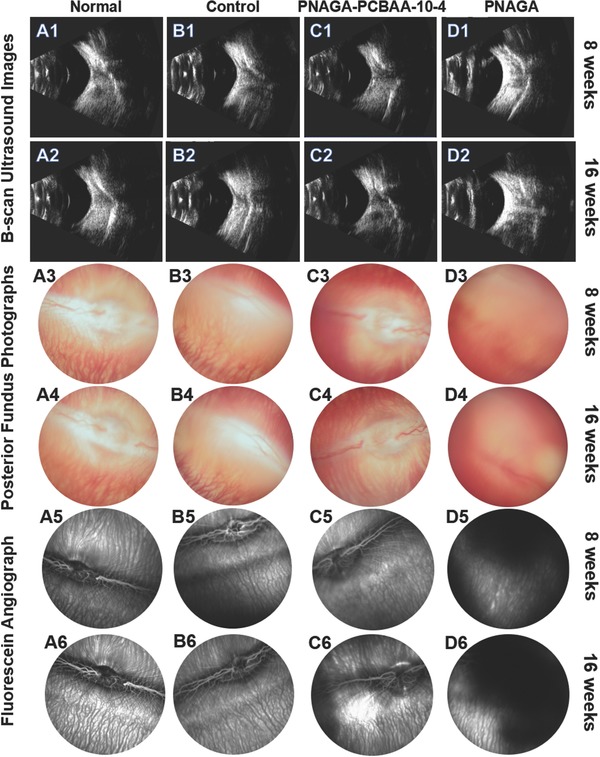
B‐scan ultrasound images, postoperative posterior fundus photographs, and fluorescein angiographs of the four groups' eyes at 8 weeks and 16 weeks post operation. A1–A6) Normal rabbit eyes. B1–B6) Sham‐operated groups. C1–C6) PNAGA‐PCBAA‐10‐4 hydrogel groups. D1–D6) PNAGA hydrogel groups.
